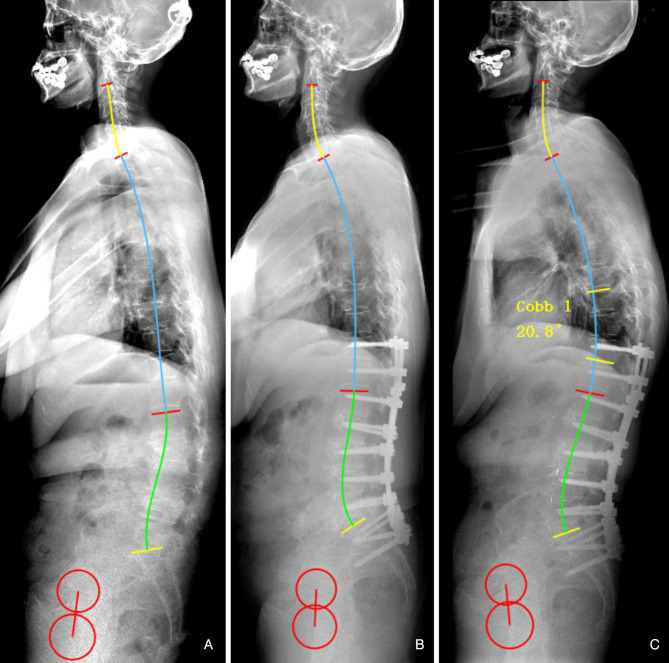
60-year-old female. (A) preoperative sagittal standing full spine X-rays, pelvic incidence (PI) = 57.3°, thoracic kyphosis (TK) = 4.4°, lumbar lordosis (LL) = −2.6°. (B) immediate postoperative X-rays, pelvic incidence = 57.7°, thoracic kyphosis = 23.8°, lumbar lordosis (LL) = −34.6°; ideal LL = 0.7*57.7 + 0.4*23.8 + 1 = −50.9°, ΔLL = 34.6–50.9 = 16.3°. (C) PJK occurred at 2-year follow-up.
